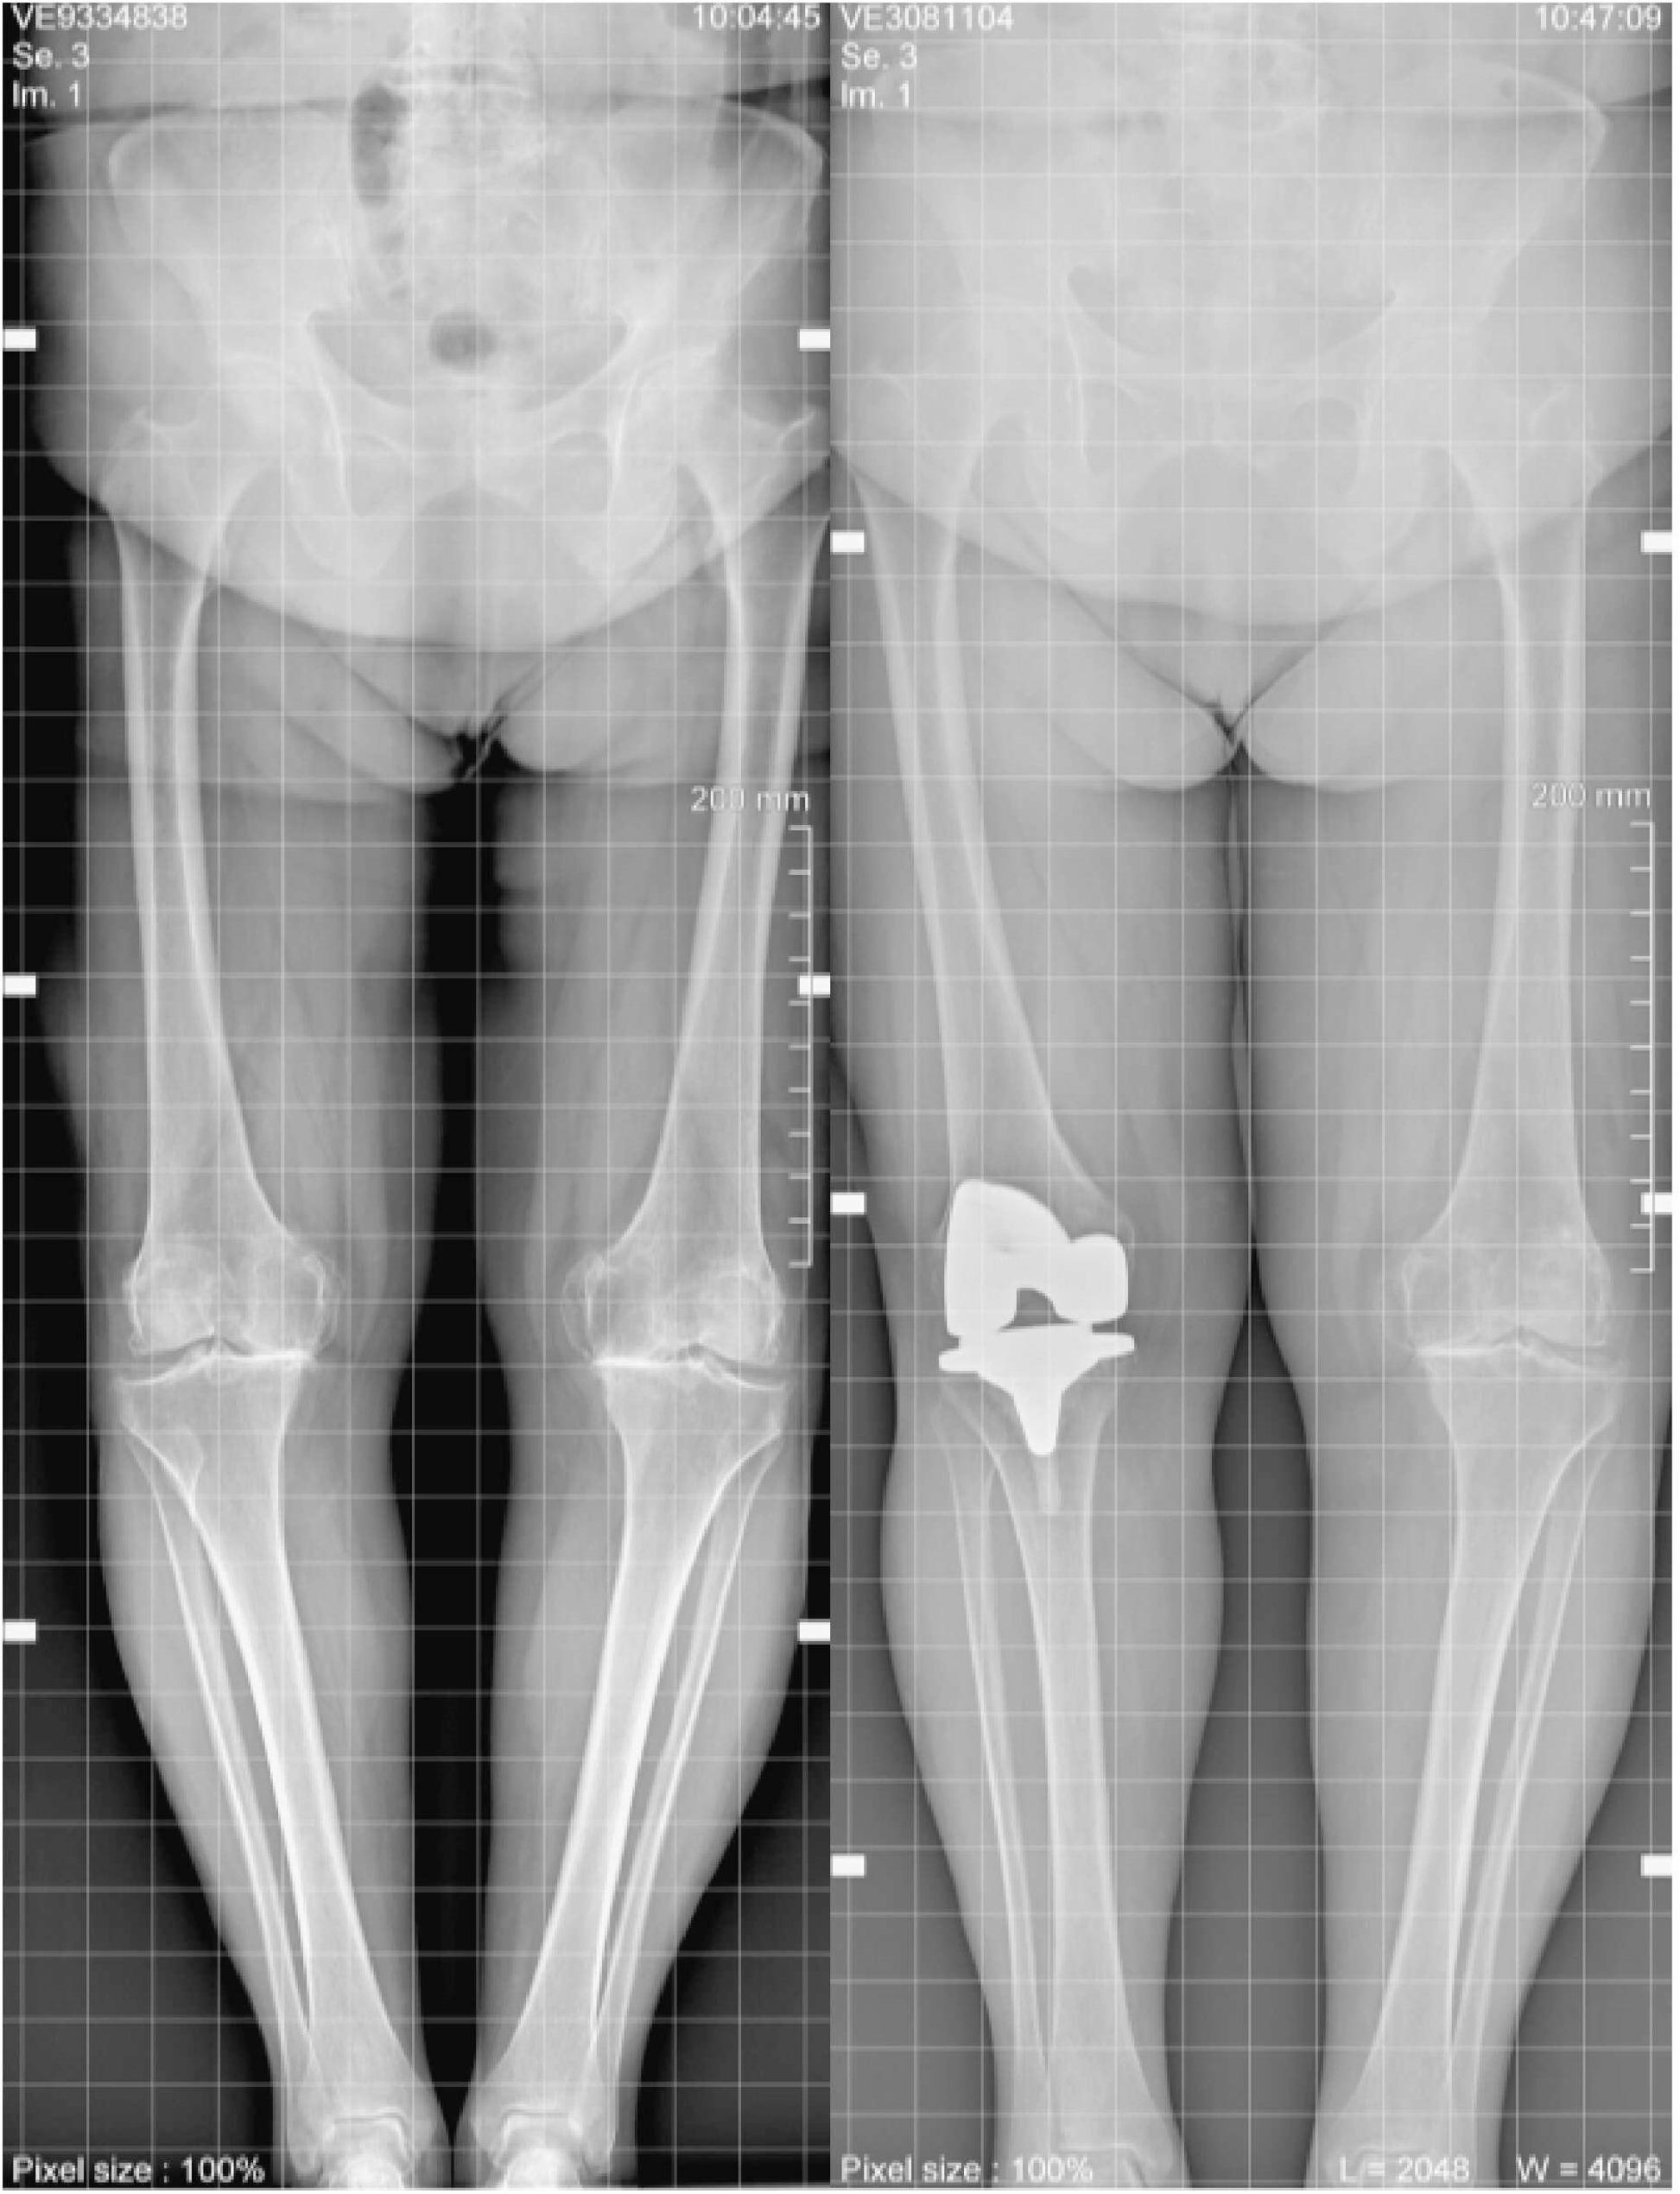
Radiographs of a female patient before surgery and 30 days postoperatively
